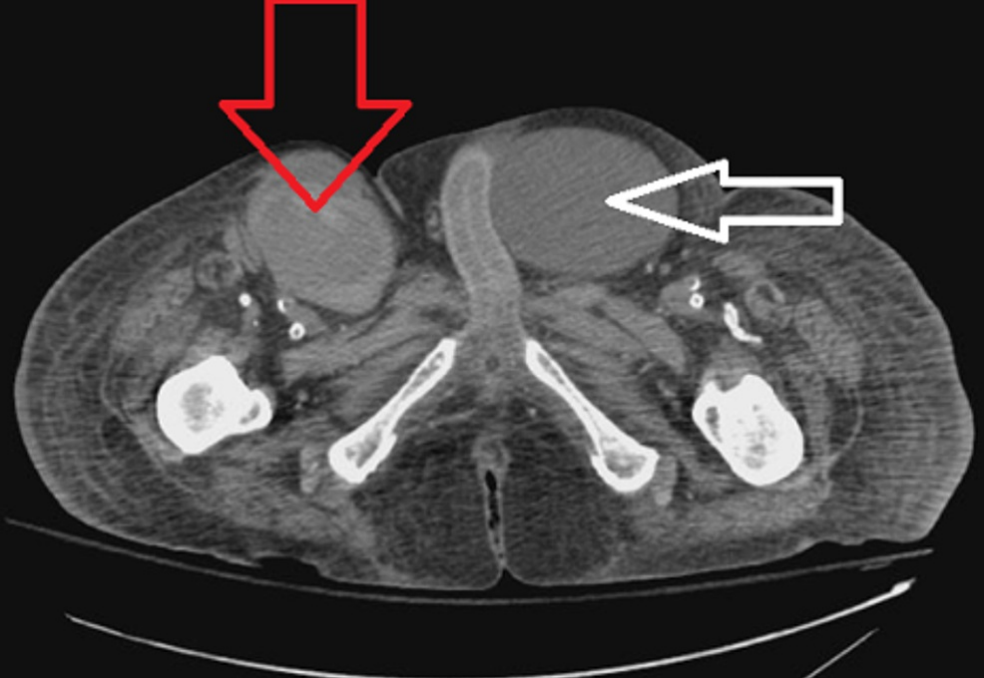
Axial CT scan without IV contrast. Red arrow is pointing at the right superficial femoral artery pseudoaneurysm. White arrow is pointing at a large inguinal hernia that was discovered concurrently.
